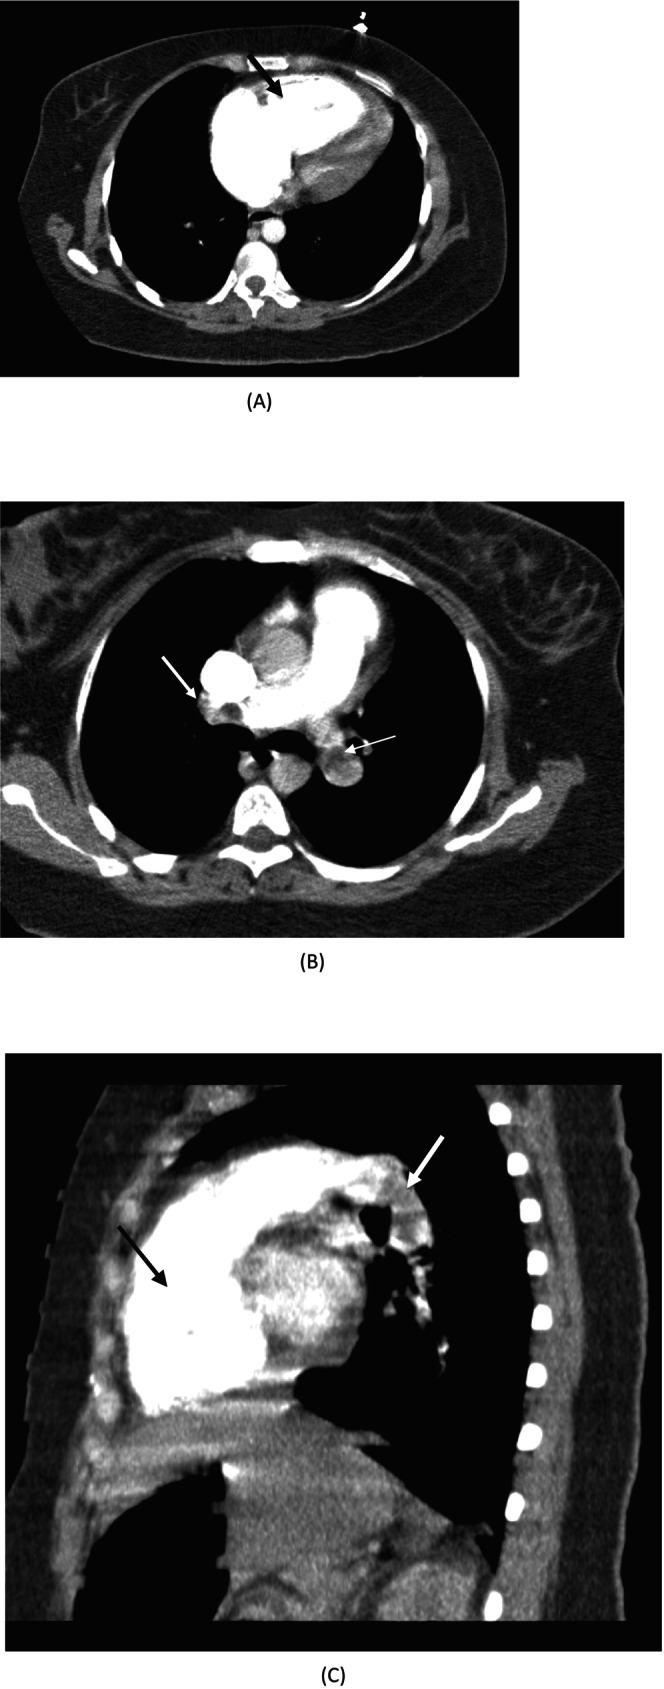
Chest CT angiography findings revealed right ventricular enlargement (black arrows in figures A&C) and pulmonary thromboembolism (white arrows in figures B&C). (A) Cross sectional view of the heart. (B) Cross sectional view of the pulmonary arteries. (C) Coronal view of the heart and pulmonary arteries.
